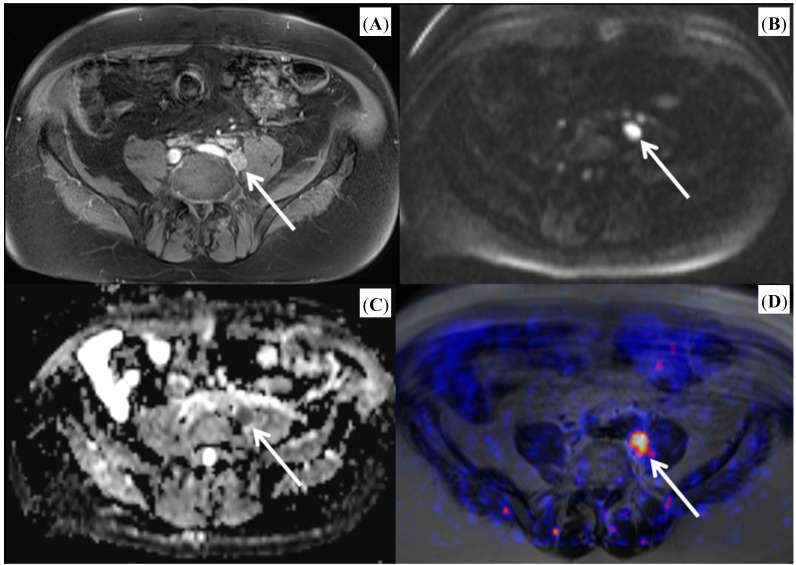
Patient with a lymph node metastasis from prostate cancer. (A) T1-weighted image after administration of contrast medium showing an enlarged left iliac lymph node with contrast enhancement; (B) Diffusion-weighted images revealing a diffusion restriction of the lymph node indicated by a hyper-intense signal; (C) Corresponding apparent diffusion coefficient (ADC) map with hypo-intense depiction of the lymph node; (D) Fused PET/MR image with pronounced choline uptake of the lymph node. (Arrows indicate lymph node metastasis).
